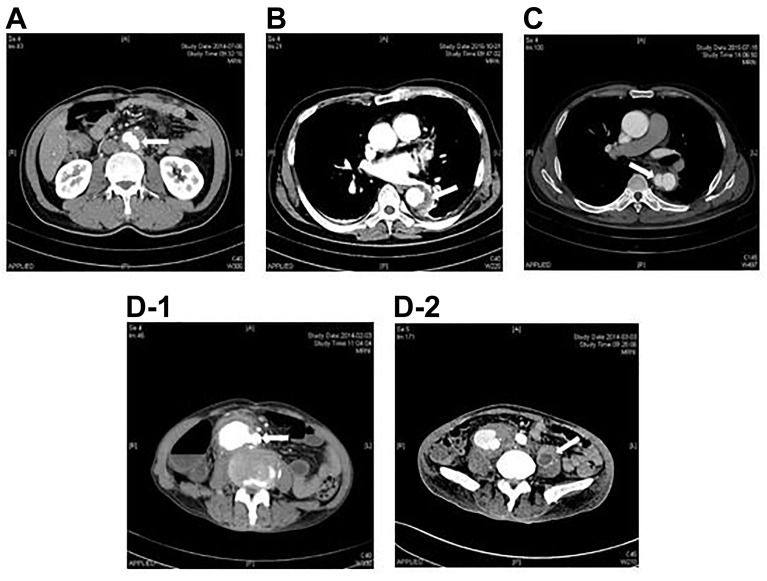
Preoperative enhancement computed tomography of the 4 patients with mycotic aneurysm (lesions: white arrows). *A*, Male, 41-year-old, CT scan of abdomen demonstrating an eccentric dilatation of the blood vessel lumen at the bifurcation of the abdominal aorta and iliac vessels. A filling defect was observed. *B*, Male, 62-year-old, CT scan of chest demonstrating an eccentric thickening of the distal segment of the thoracic aorta accompanied by calcified intimal displacement. *C*, Male, 75-year-old, CT scan of chest demonstrating a saccular aneurysm formation at the thoracic segment of descending aorta and mural filling defect. *D-1,* Male, 67-year-old, CT scan of abdomen showing pseudoaneurysm formation at the abdominal aortic bifurcation and the initial segment of the right iliac artery. *D-2*, Same patient, showing the formation of the left psoas abscess.
